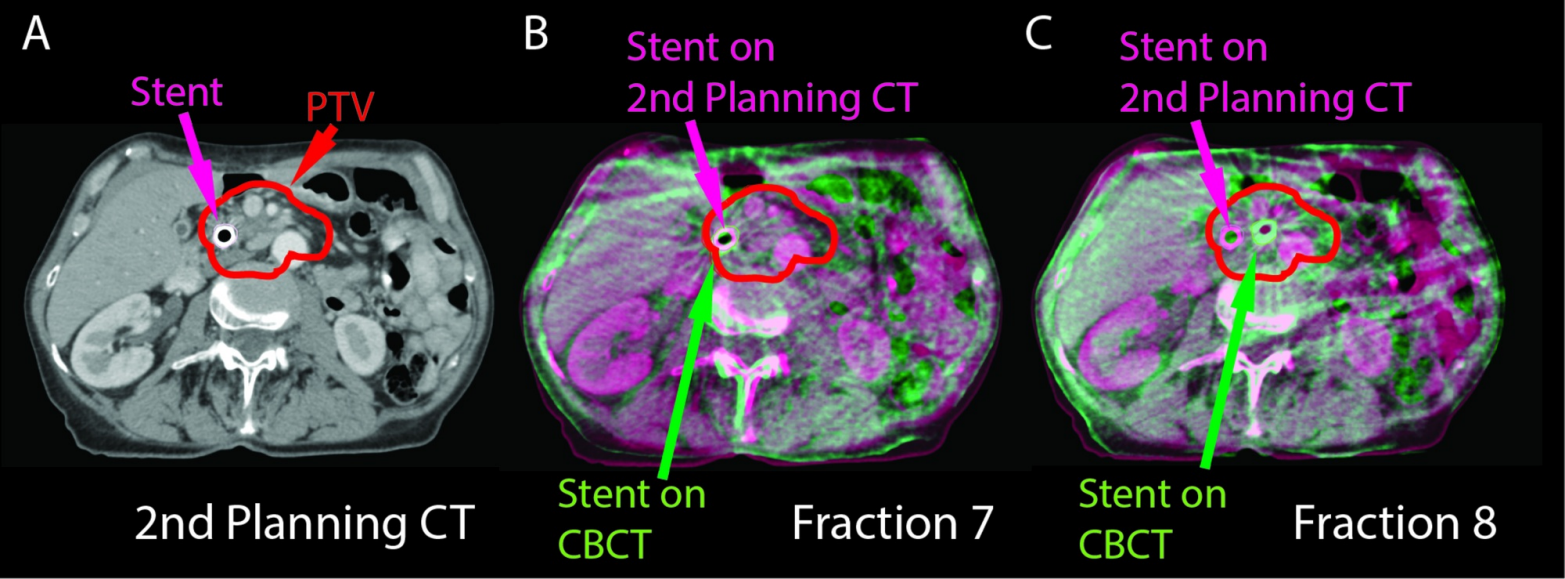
CT and CBCT fusions. Second planning CT (A) and fused planning CT with CBCTs are shown (B-C). The planning CT is in purple and the CBCT is in green. Although the stents were aligned on fraction 7 (B), on fraction 8 the stent moved 20 mm medially with maintained vertebral body alignment (C). PTV: Planning target volume; CBCT: Cone-beam computed tomography.
